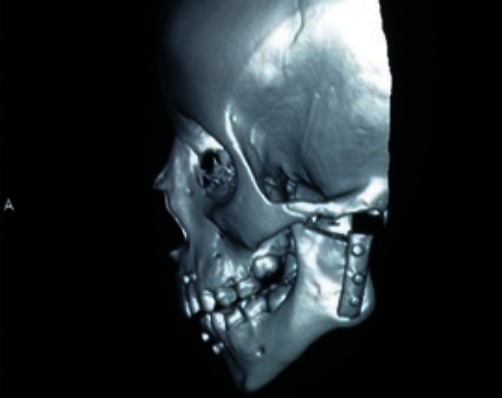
A post-operative 3-D reformatted image of a CT scan showing the graft in place.
